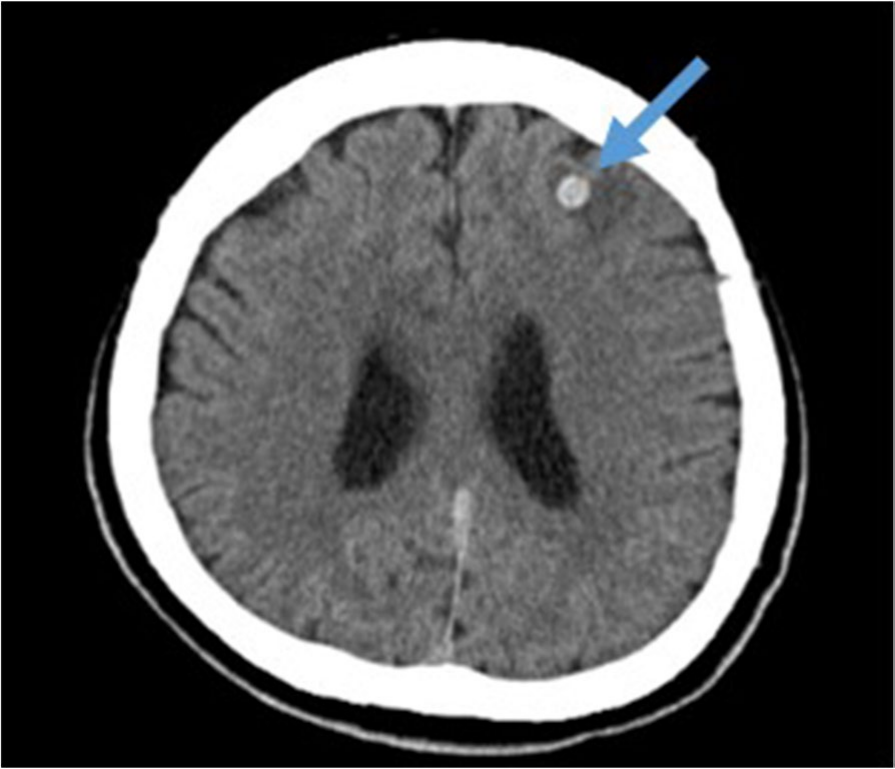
Postoperative brain CT scan. Postoperative brain CT scan 2 h after extubation shows only a high-density lesion surrounded by a low-density area in the left frontal lobe (blue arrow). The lesion was presumed to be old bleeding before further investigation. CT, computed tomography
